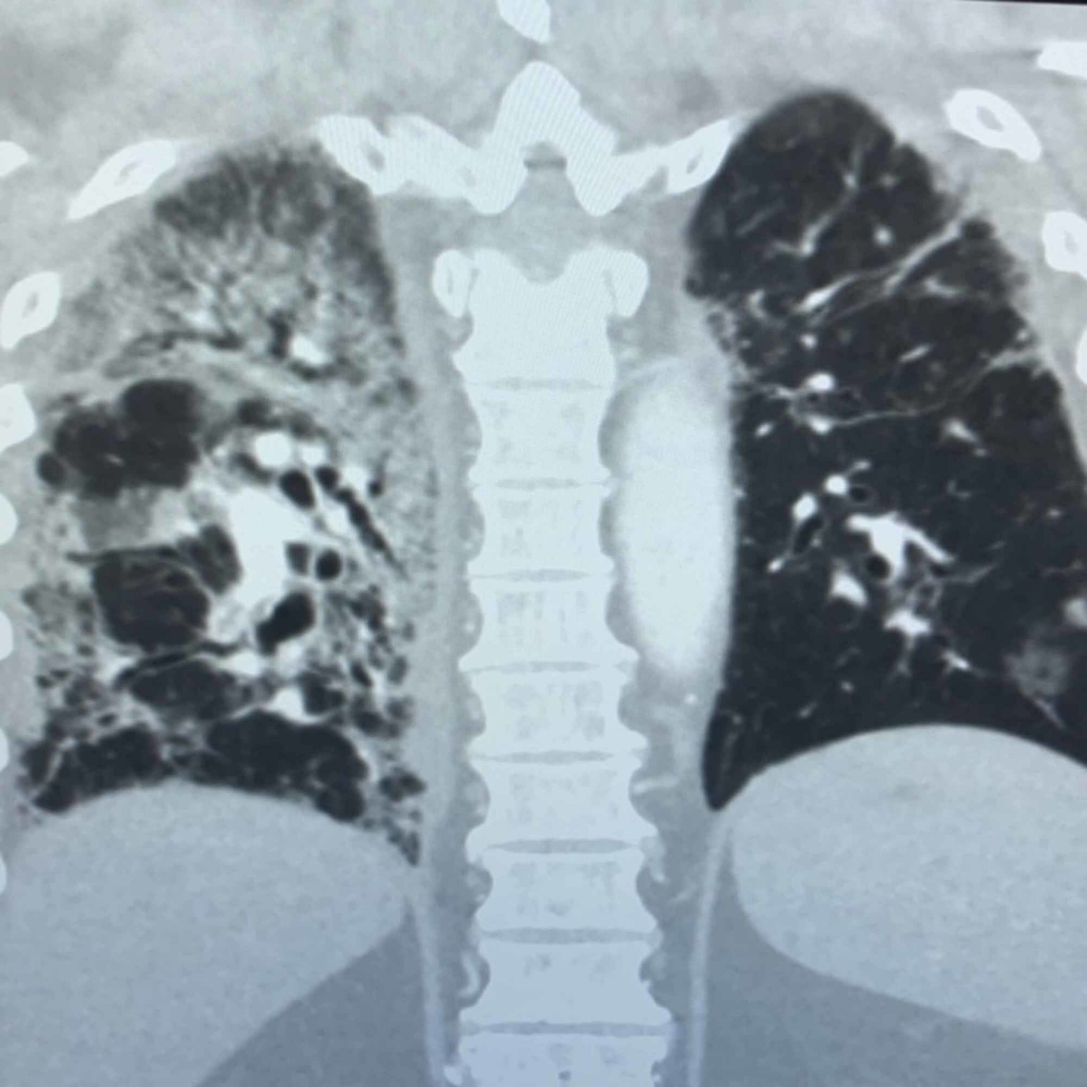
Coronal chest CT showing ground-glass opacities superimposed on diffuse fibrotic changes of the lung parenchyma with traction bronchiectasis in both upper and lower lobes CT: computed tomography
